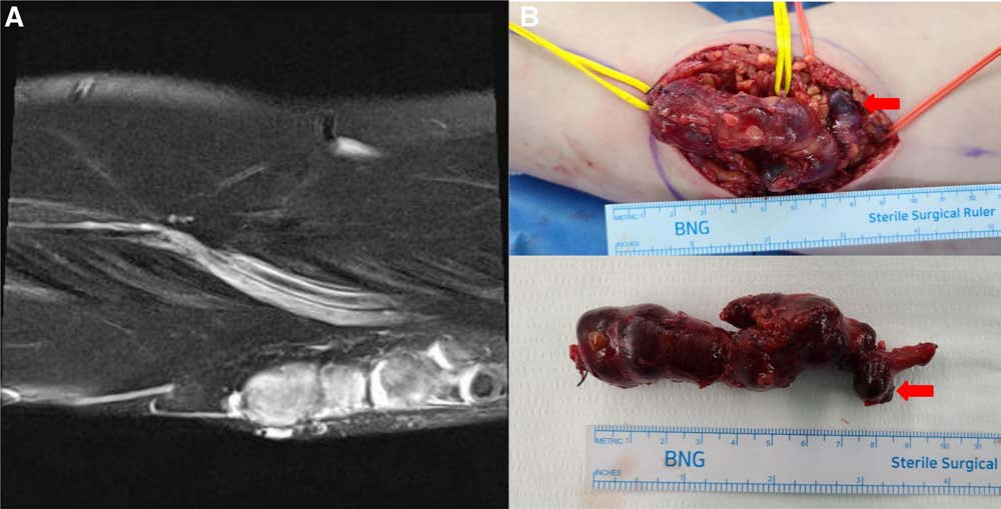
Preoperative radiograph (A) and intraoperative photographs (B) of right upper arm. (A) Magnetic resonance image of the upper arm shows venous enlargement with a thrombus in the basilic vein. (B) Operative findings that intravascular myopericytoma caused occlusion and venous enlargement by narrowing the proximal basilica vein (red arrow).
